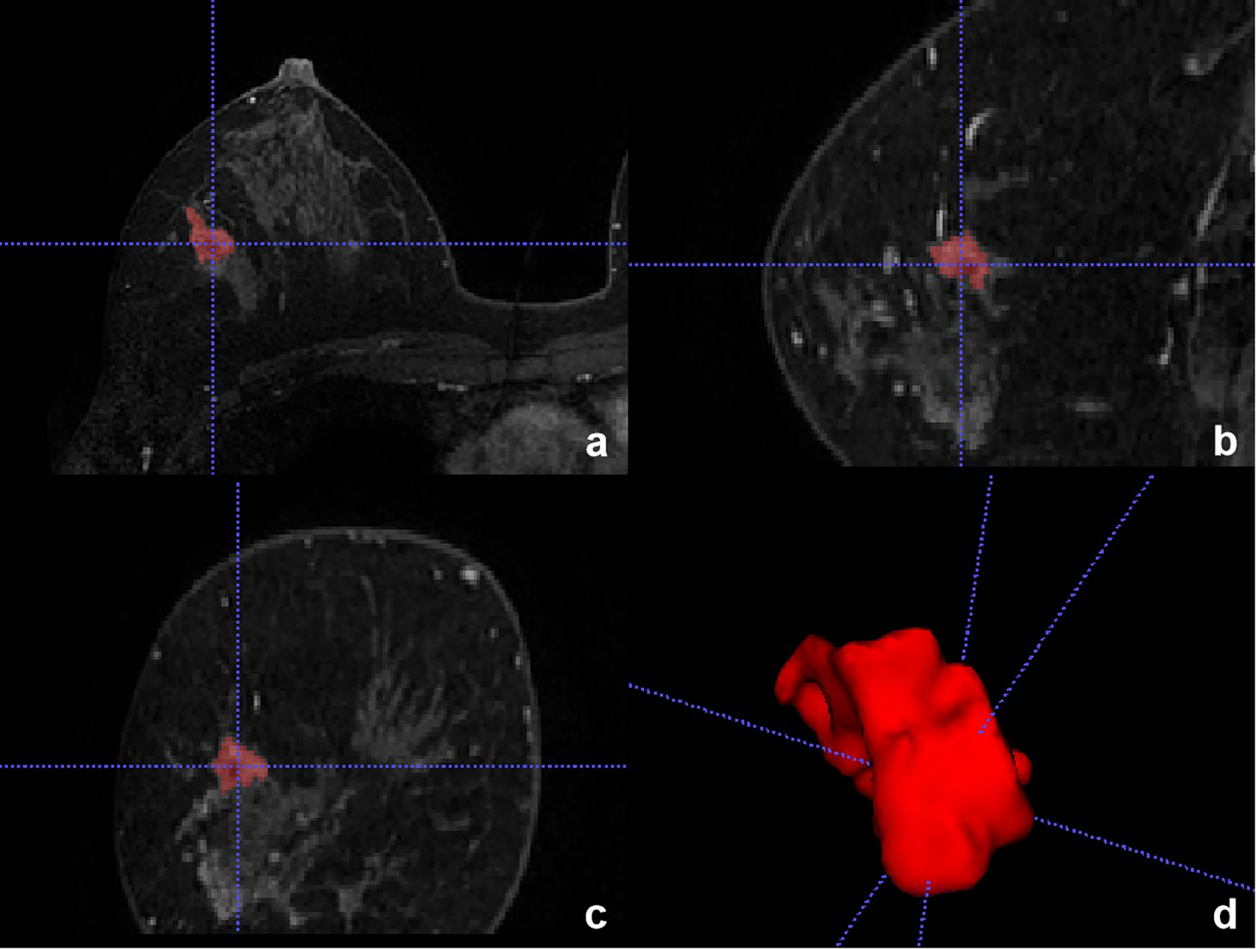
Adenosis in a 46-year-old woman in the testing set with false-positive result. (A–C) Axial, Sagittal, and Coronal T1-weighted postcontract image showed non-mass enhancement in the right breast. (D) 3D reconstruction of the lesion.
